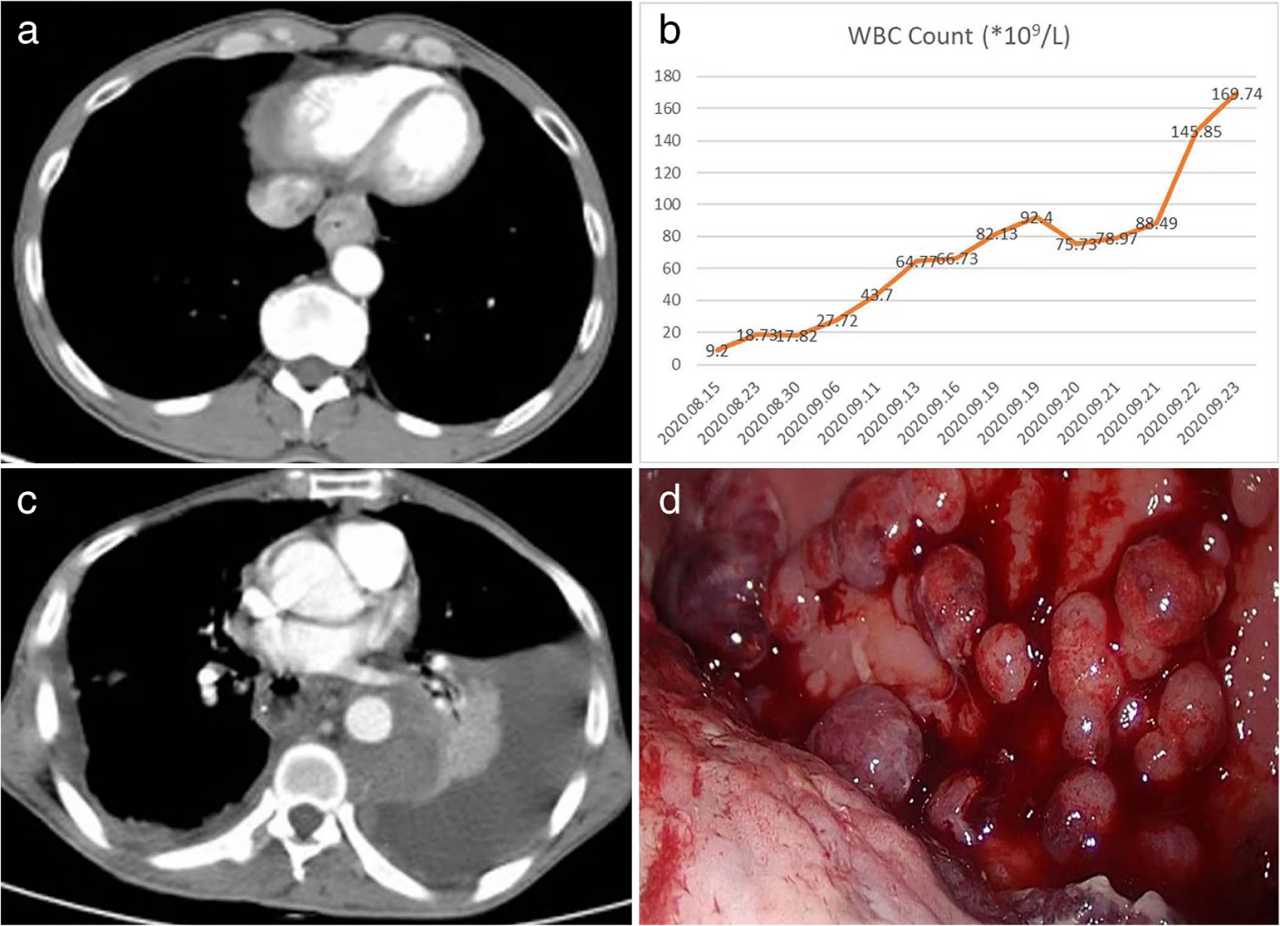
(a) Chest computed tomography (CT) revealed a mass in the lower part of the esophagus. (b) Perioperative tendency of the WBC. (c) Chest CT scans 28 days after the surgery revealed soft tissues around the descending aorta, massive pleural effusion in the left thoracic cavity, and numerous pleural nodules. (d) Thoracoscopy revealed pleural dissemination with nodules in the left thoracic cavity
